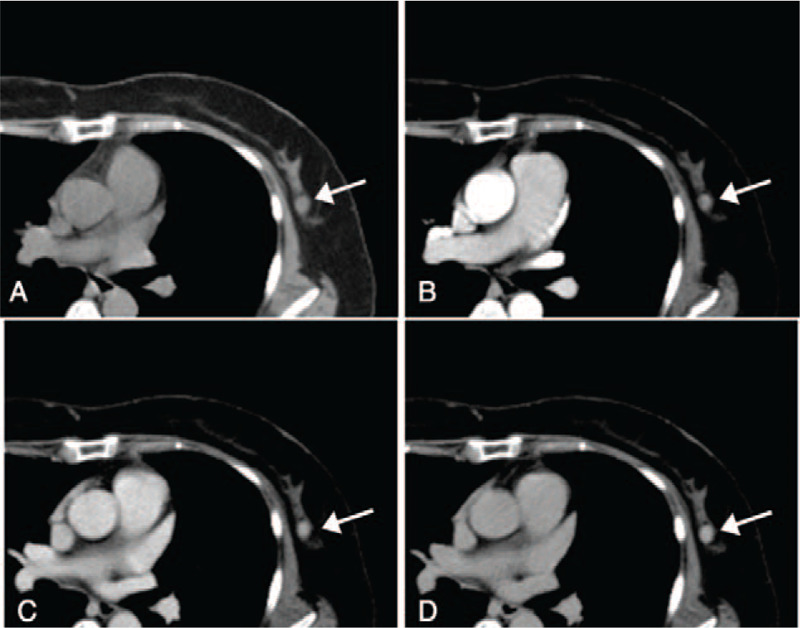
The dynamic CT image obtained 3 years before shows a pattern of gradual increase (arrows), which is known as the benign pattern. The mass presents as a homogeneous tumor with defined margin showing 30–33 Hounsfield Unit (HU) on non-contrast CT (a) and that increases to 87–92 HU in the arterial phase (b), 109–116 HU in the venous phase(c), and 119–123 HU in the capillary phase (d).
